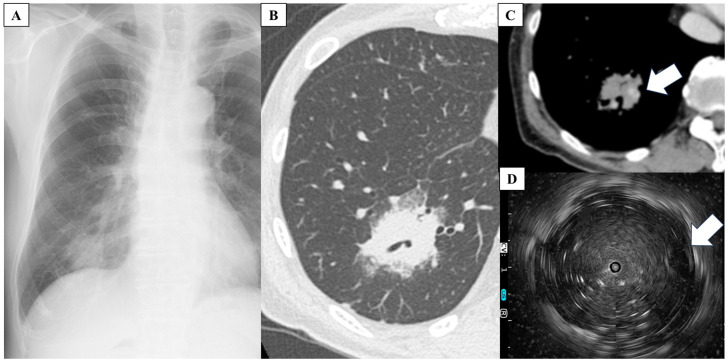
(A) Chest radiograph shows a mass in the right lower lung field. (B,C) High-resolution computed tomography of the chest shows a 30 mm mass with a cavity in the posterolateral lower lobe of the right lung with a bronchial artery (white arrow) inside. (D) Endobronchial ultrasonography shows the bronchial artery (white arrow).
